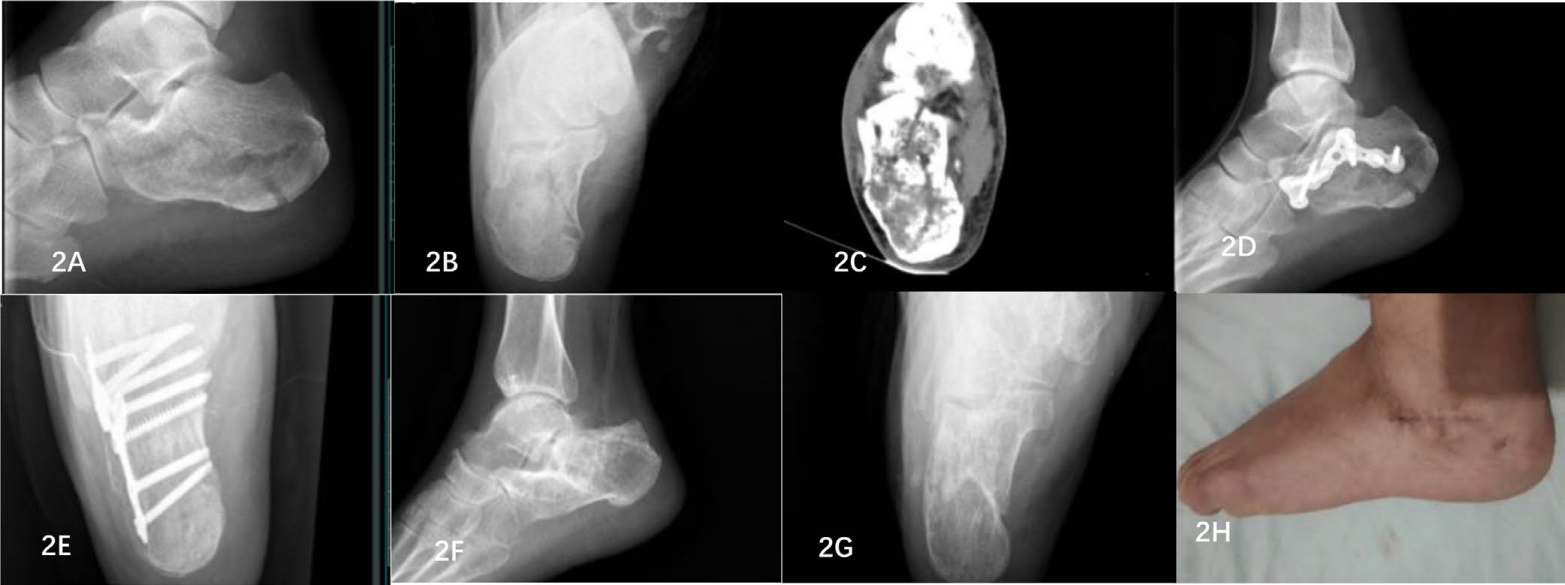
Imaging data of a typical TSIPIF patient. (A) Preoperative calcaneal lateral x‐ray image showing that the articular surface of the calcaneus collapsed. (B) Preoperative axial x‐ray of the calcaneus revealed that the width of the calcaneus increased and that the length of the calcaneus shortened. (C) Preoperative MR image of the calcaneal fracture suggested a Sander type III fracture. (D) Postoperative calcaneal lateral x‐ray image showing calcaneal height recovery. (E) Postoperative calcaneal axial x‐ray image showing that the width and length of the calcaneus had recovered. (F) Lateral x‐ray films of calcaneus after removal of internal fixator showed calcaneus recovery. (G) Axial x‐ray films of calcaneus after removal of internal fixator showed calcaneus recovery. (H) The wound healed well after the operation.
